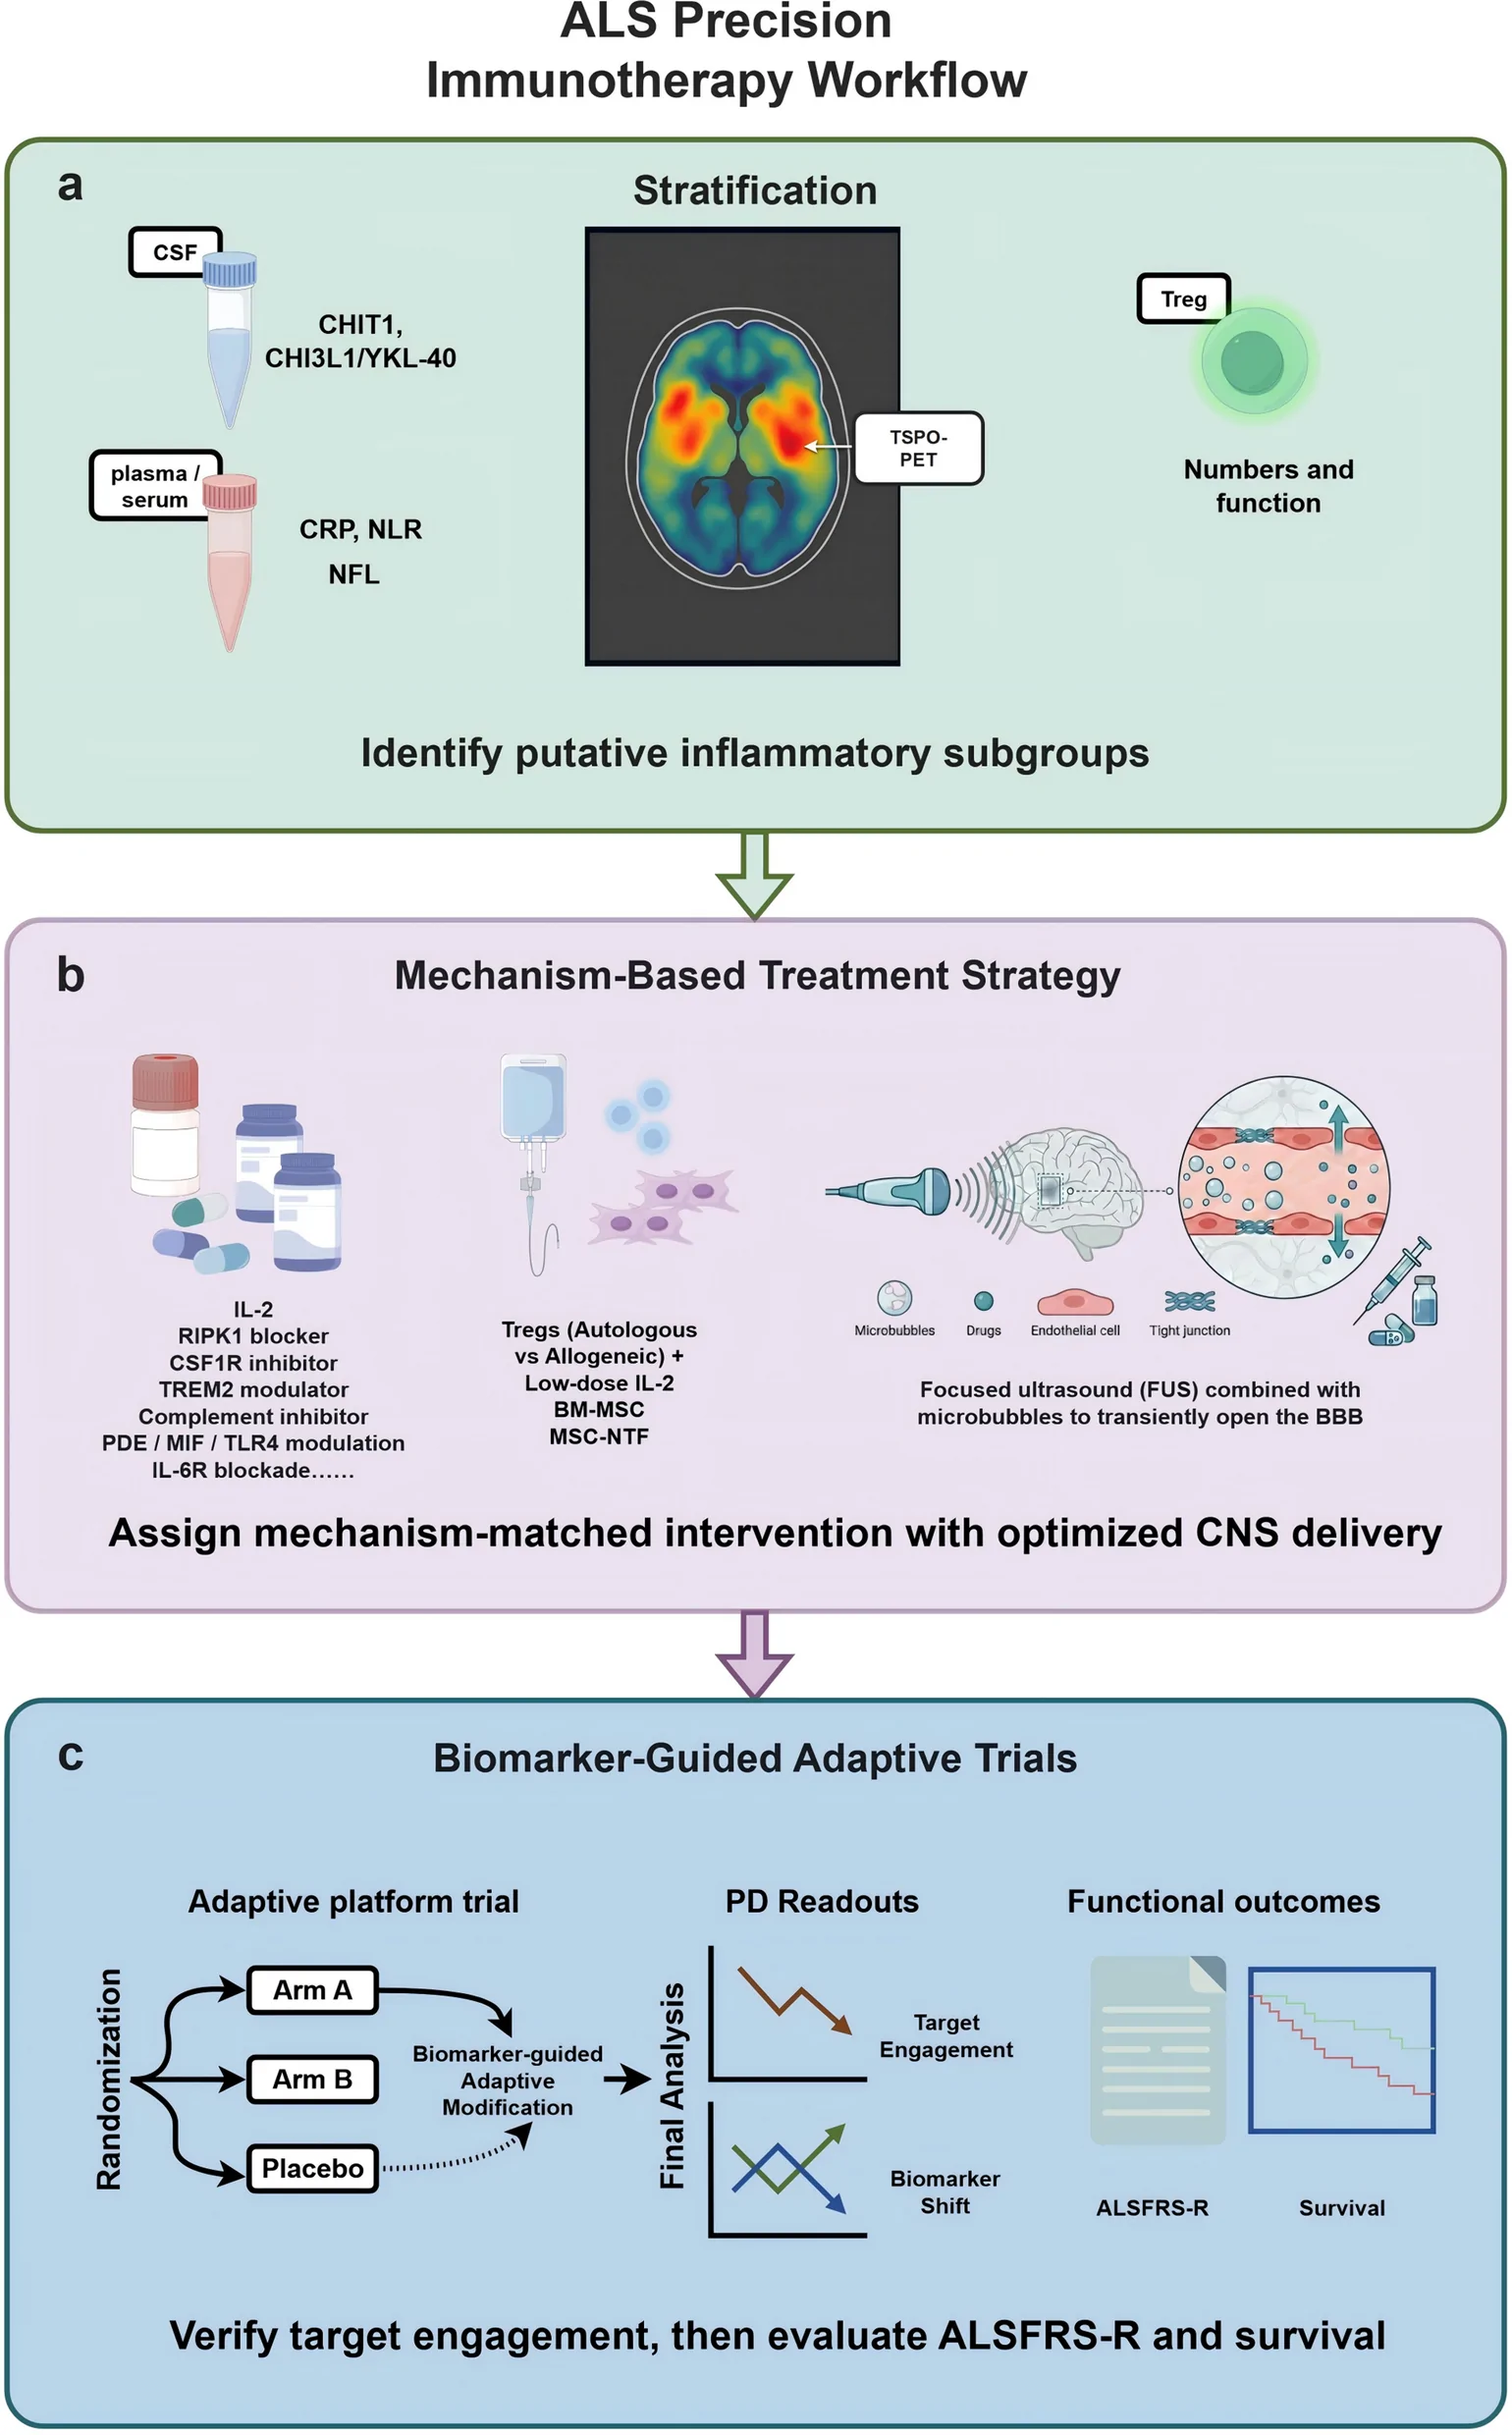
Precision immunotherapy workflow for ALS. A proposed three-stage framework for precision immunotherapy in ALS. a Stratification. Patients are profiled using a multimodal biomarker panel to identify putative immune subgroups. CSF markers (CHIT1, CHI3L1/YKL-40) reflect CNS glial activation; plasma markers (CRP, neutrophil-to-lymphocyte ratio [NLR], NfL) capture systemic inflammation and axonal injury; TSPO-PET provides spatial quantification of glial activation in the motor cortex and spinal cord; and Treg cell numbers and suppressive function complete the peripheral immune profile. b Mechanism-based treatment strategy. Candidate interventions are selected according to the dominant immune mechanism identified in Stage a. Investigational agents include small molecules and biologics targeting innate immunity (RIPK1 blockers, CSF1R inhibitors, TREM2 modulators, complement inhibitors, IL-6R blockade) and adaptive immunity (low-dose IL-2). Cell-based approaches include Treg infusion and MSC-derived therapies. Focused ultrasound (FUS) with microbubbles provides spatially targeted BBB opening to enhance CNS drug delivery. c Biomarker-guided adaptive trial. Pharmacodynamic readouts regarding target engagement and biomarker shifts, are verified before interpreting functional outcomes (ALSFRS-R, survival), enabling distinction of biological failure from pharmacokinetic or patient-selection failure
